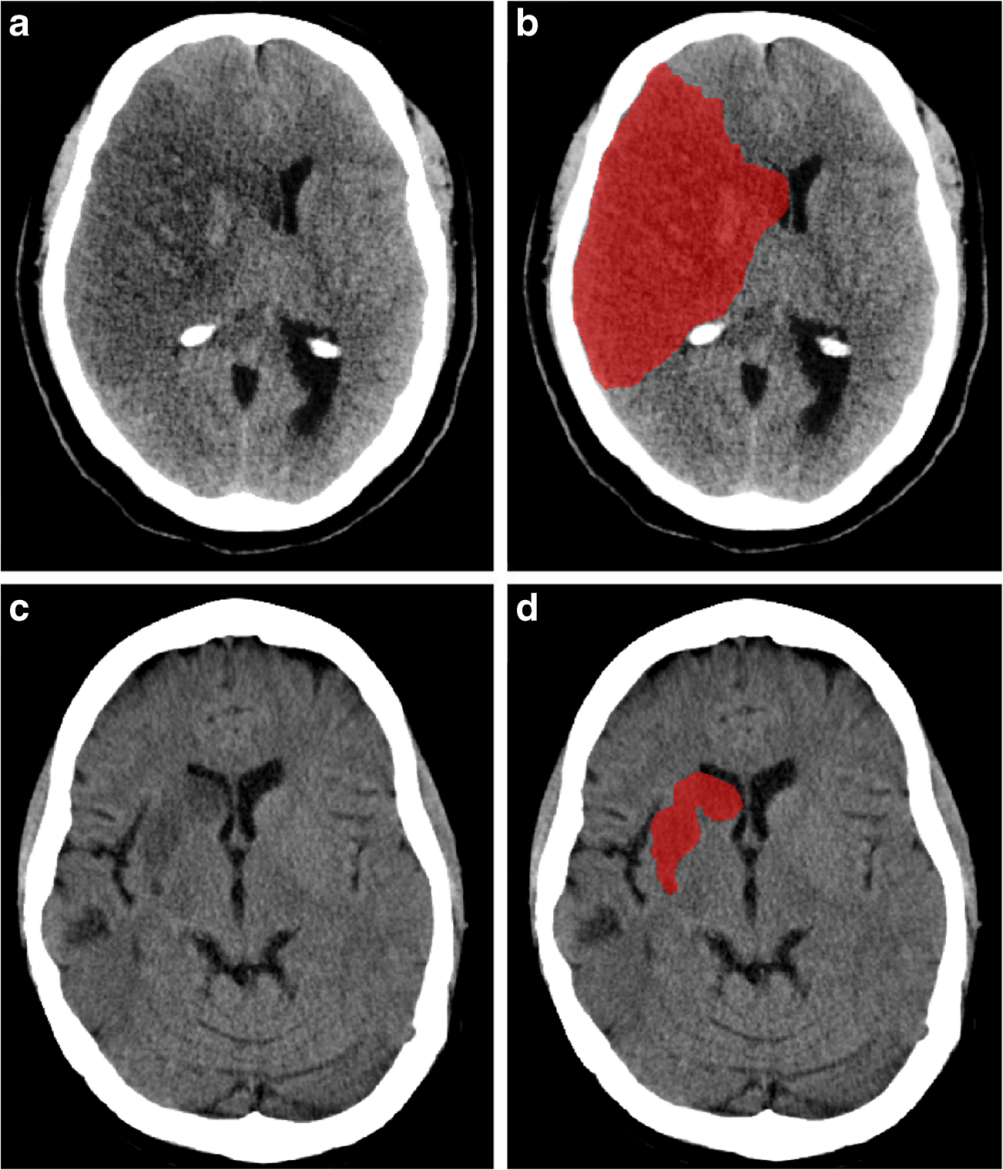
Case examples of follow-up infarct (FIV) segmentation on non-contrast CT, acquired between 5 and 7 days after onset. (a and b) A 56-year-old male with right-sided M1 occlusion. FIV was 292 ml and this patient was severely disabled at 90 days (mRS 5). (c and d) A 45-year-old female with right-sided M1 occlusion. FIV was 10 ml and the patient showed no significant disability at 90 days, despite some symptoms (mRS 1)
